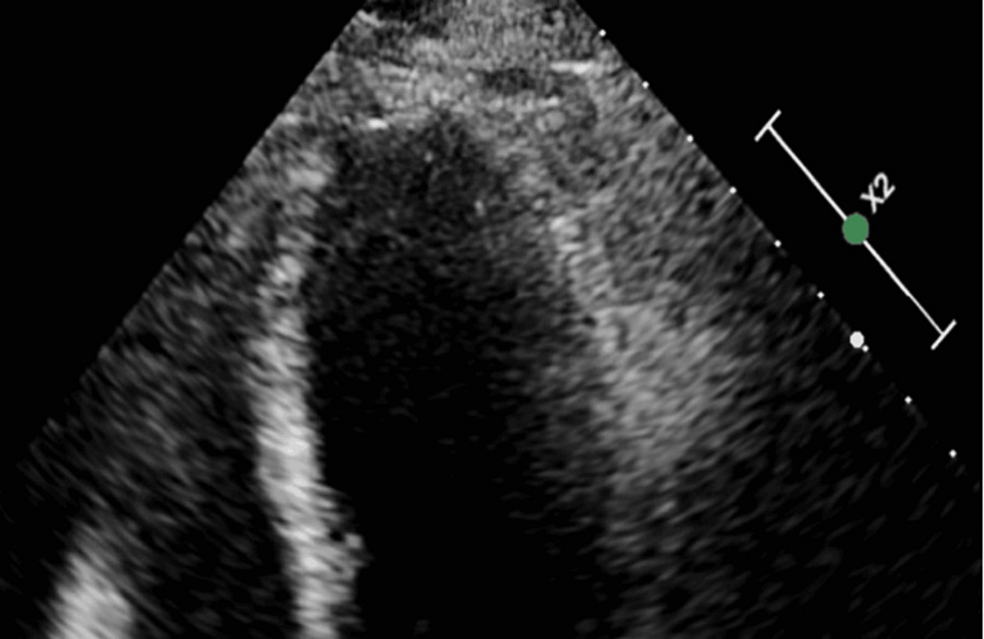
Echocardiogram with apical ballooning because of the anaphylaxis
